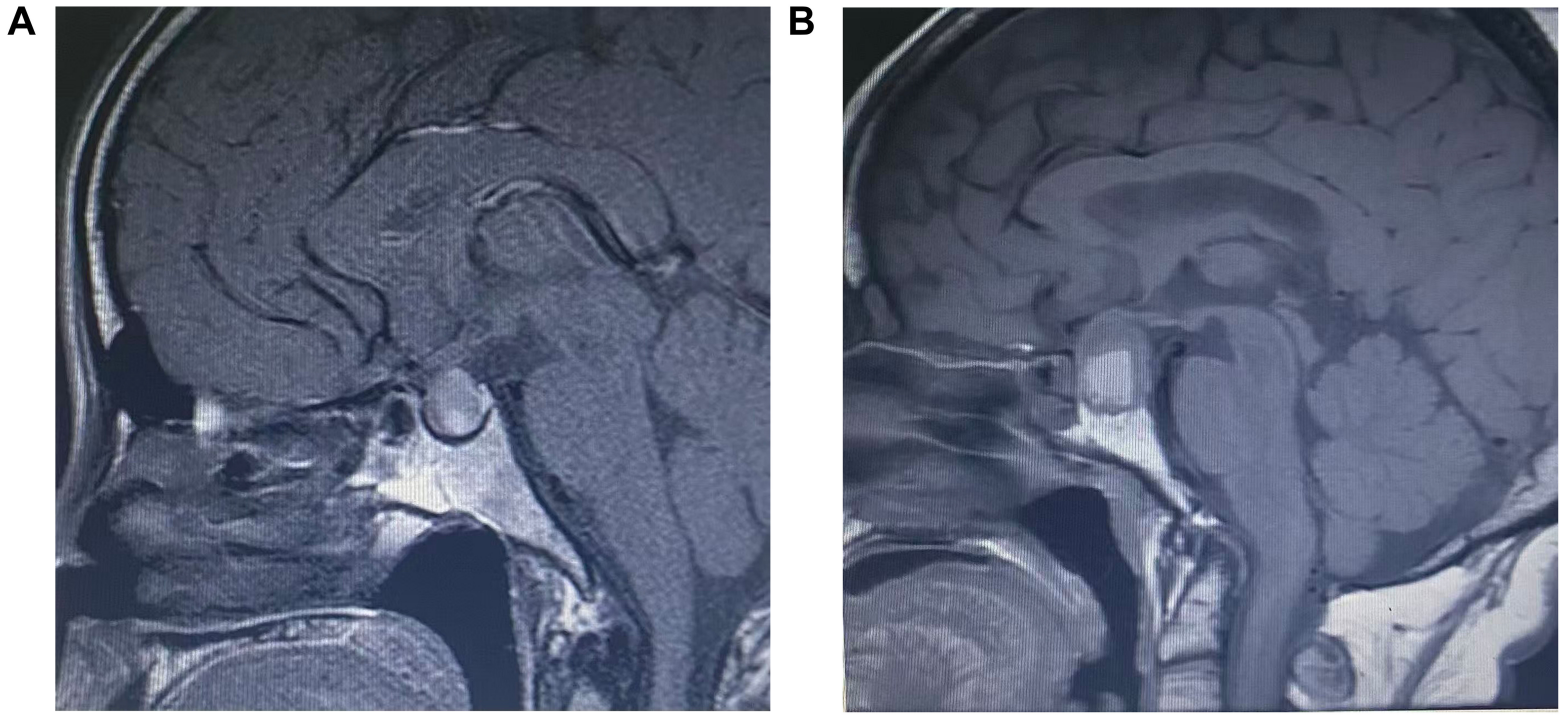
Acute pituitary apoplexy (A) and subclinical pituitary apoplexy (B) The images show the presentation of acute pituitary apoplexy (A) and subclinical pituitary apoplexy (B) on unenhanced T1-weighted images (T1WI), where the intrasellar mass shows hyperintensity, forming a signal level related to acute/subacute bleeding. T1WI: T1-weighted imaging.
